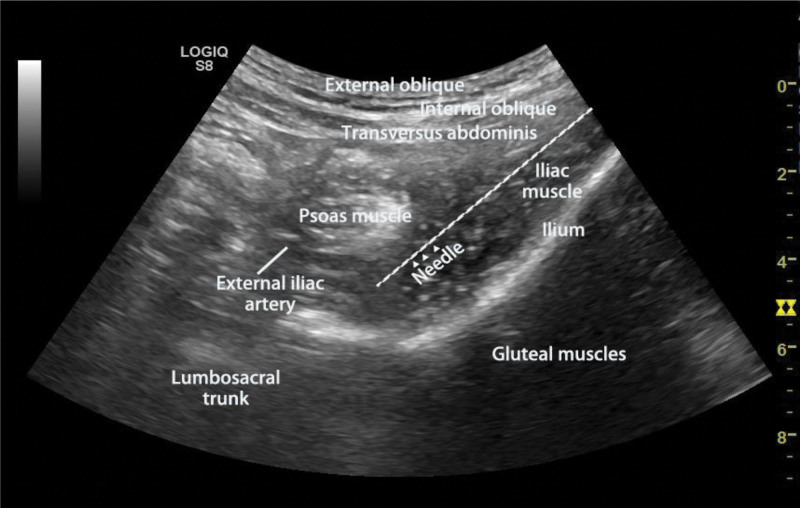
Ultrasonic image of anterior iliopsoas space block. EIA = external iliac artery, IM = iliac muscle, PM = psoas muscle.
